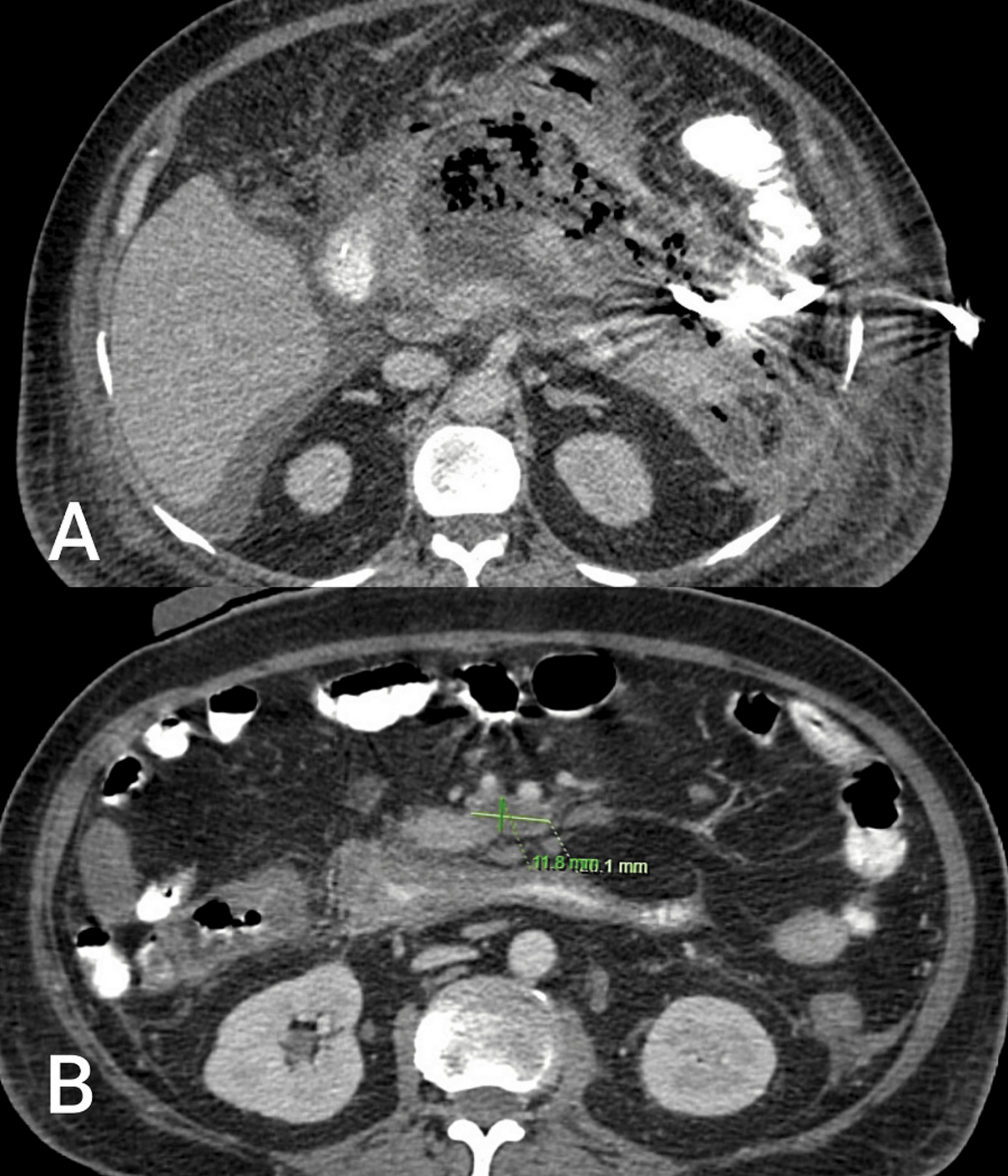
CT scan images of necrotic pancreatic collection (A) Before nephroscopic-assisted debridement; (B) After nephroscopic-assisted debridement
